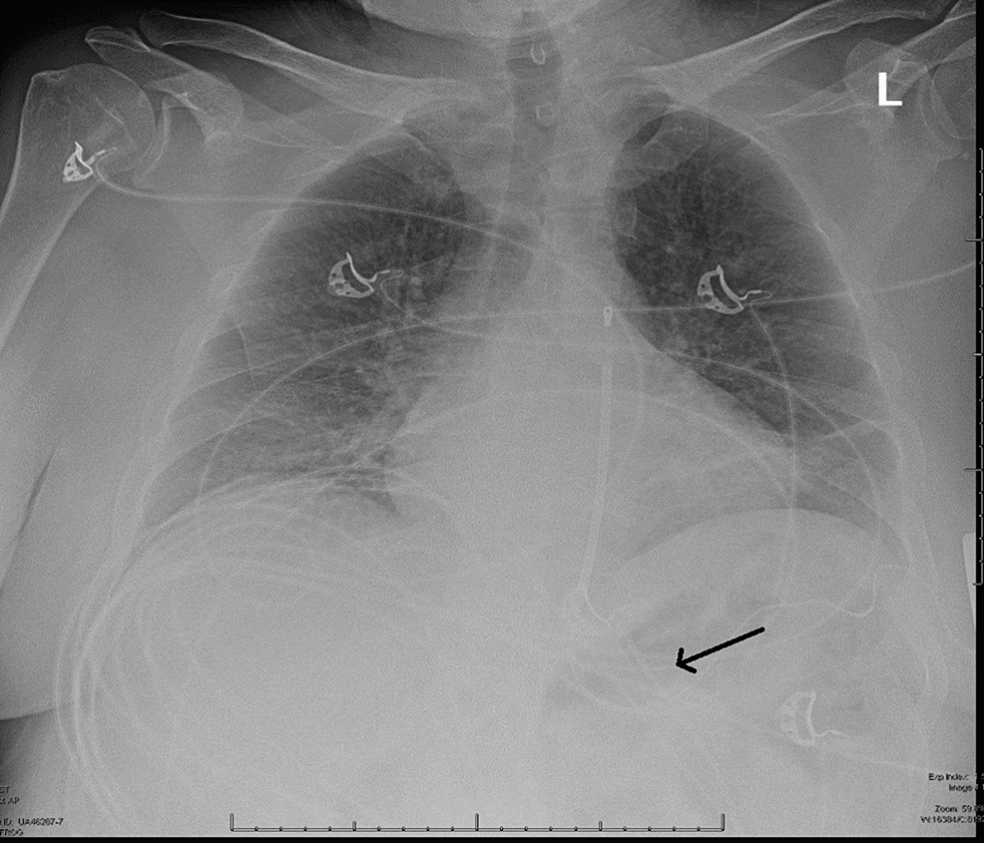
Initial chest radiography on presentation showing mild pulmonary edema and displaced S-ICD lead (black arrow). S-ICD: subcutaneous implantable cardioverter-defibrillator
